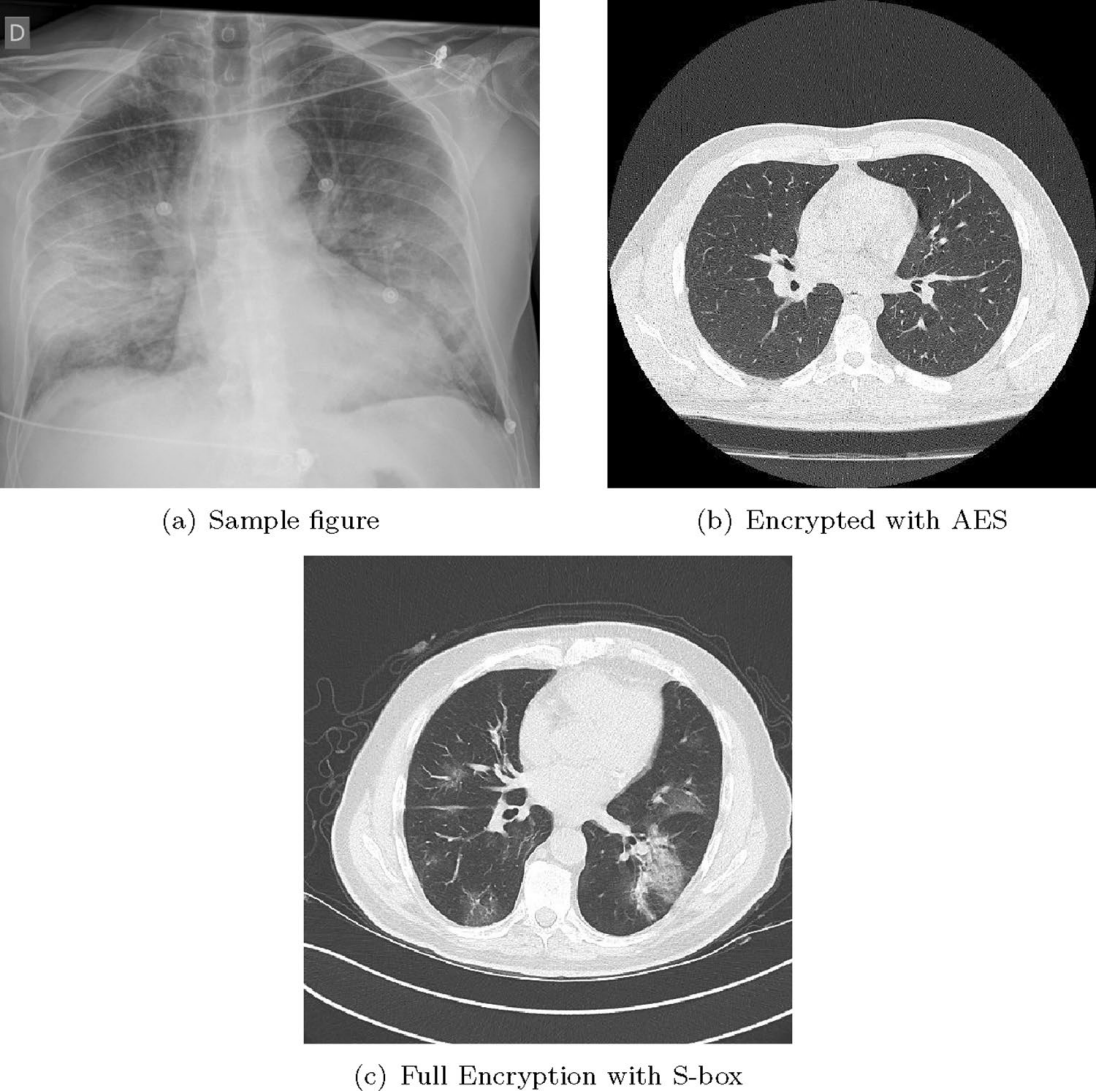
CXR and CT images of COVID-19 patients
